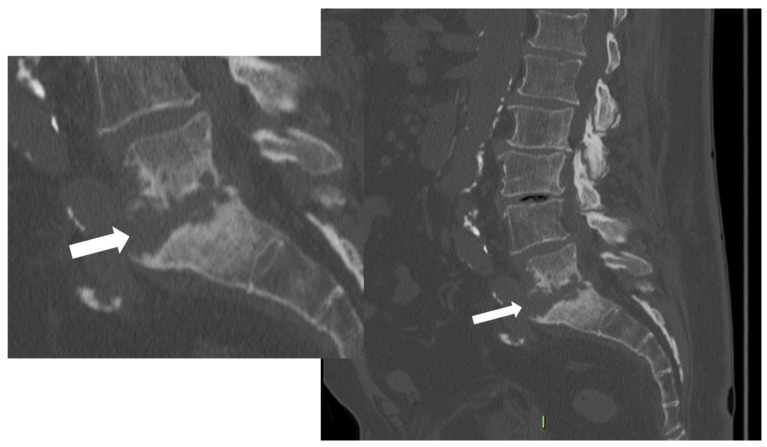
CT, sagittal reconstruction (magnification on the left), of a 77-year-old female with L5-S1 pyogenic spondylodiscitis characterized by thick endplates erosions (arrows).
